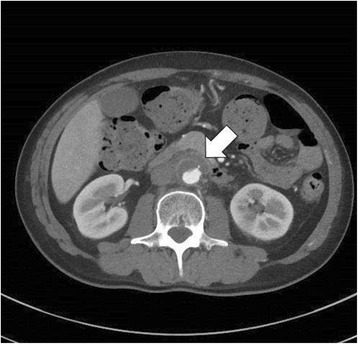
Preoperative CT scan. Axial view showing a small sacciform infrarenal abdominal aortic aneurysm (*white arrow*)
